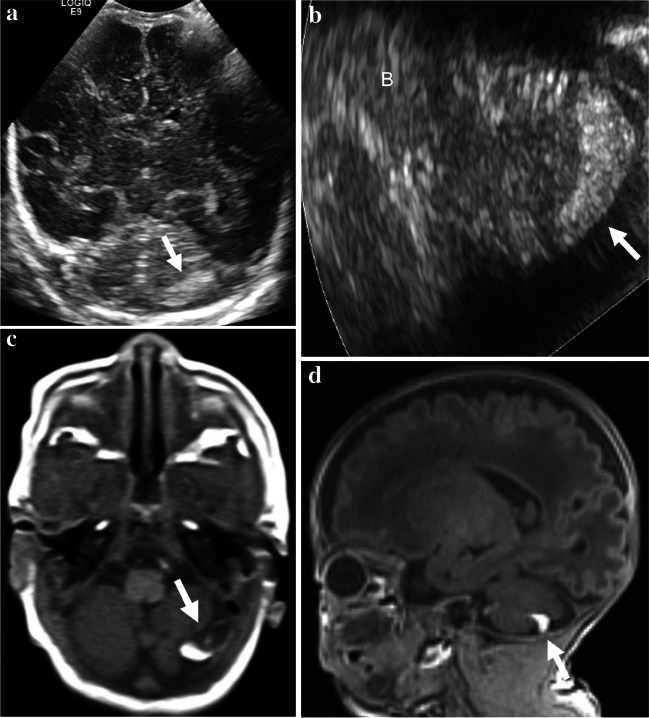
Representative case of type 3 cerebellar hemorrhage in a preterm boy (27 + 6 weeks, 880 g). a, b Oblique coronal anterior fontanelle view (a) and axial left mastoid view (b, rotated 90° clockwise to orient with the axial magnetic resonance image) of cranial ultrasound images show ovoid-to-crescent echogenic lesion (*arrows*) at the inferior periphery of the left cerebellum. c, d Unenhanced axial (c) and sagittal (d) T1-weighted magnetic resonance images obtained 7 weeks after the ultrasonography show T1-hyperintense hemorrhage (*arrow* in d) with focal cystic change (*arrow* in c)
